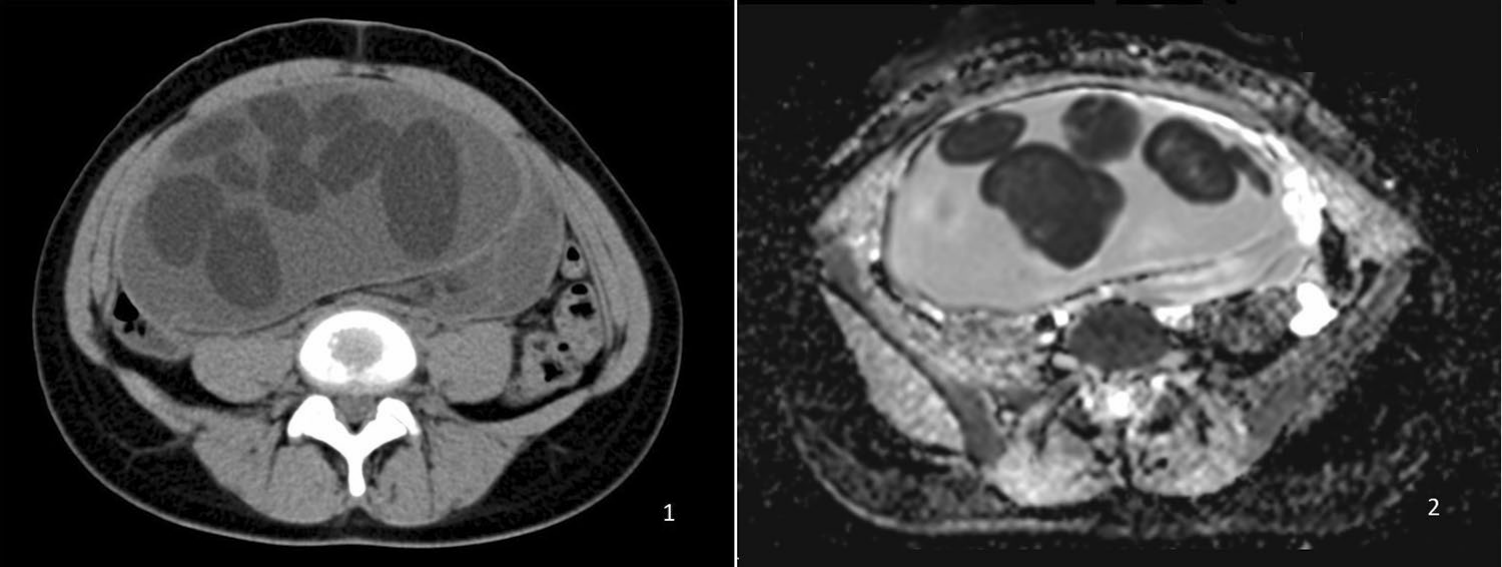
Floating balls visible in MRI (1) and CT (2) scans of a 17-year-old patient with an ovarian mature teratoma tumor
